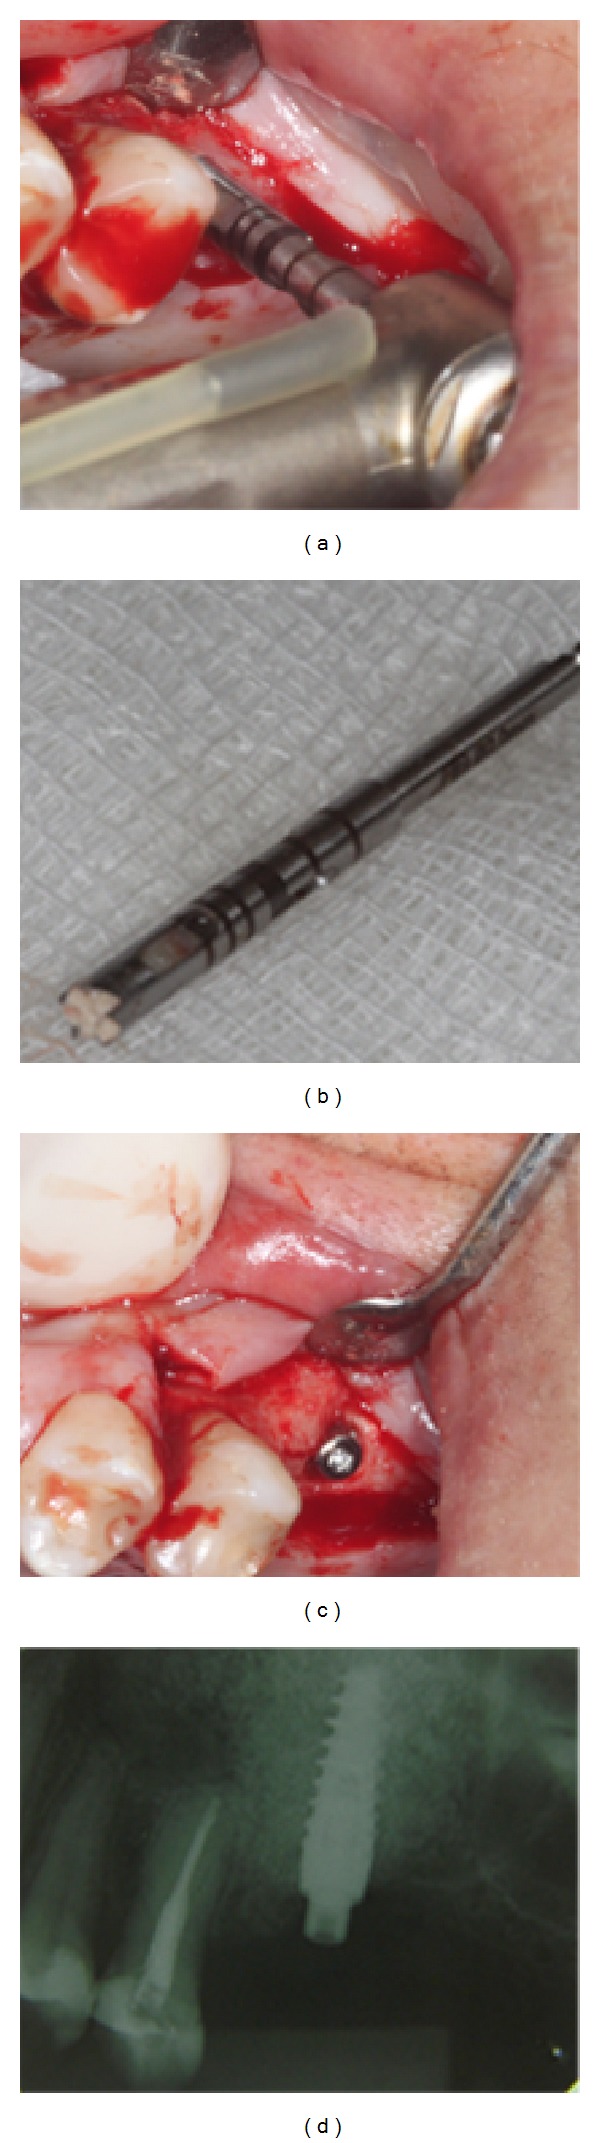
Removal of a fragment of bone tissue from the graft area (a) with a trephine number 2 bur following treatment (b). Implant placement (c) and final periapical radiograph (d).
